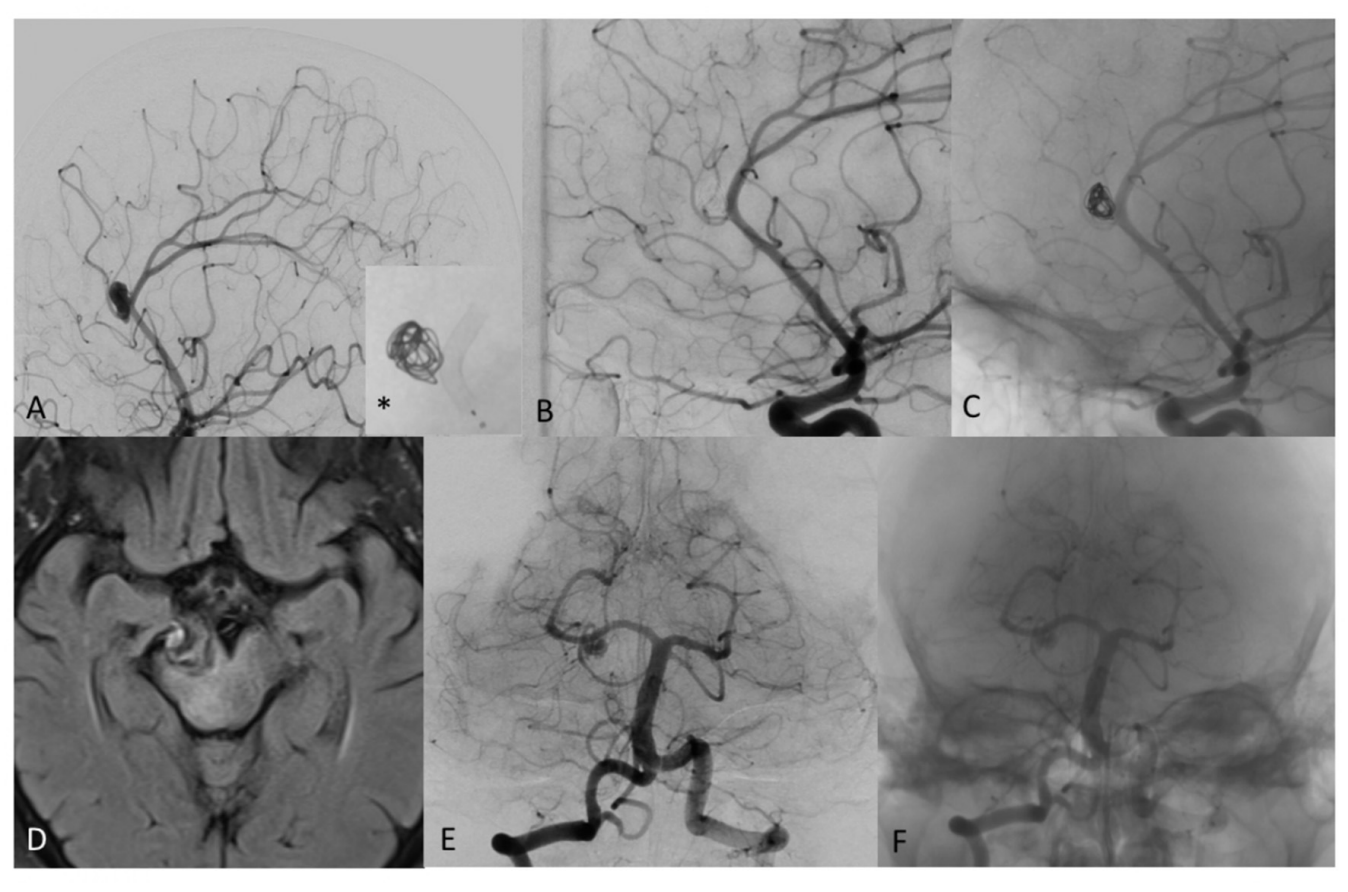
(A) Digital subtraction angiography (DSA) of a patient with distal pericallosal-callosomarginal aneurysm treated with non-compact coiling and FD (*), to maintain the patency of callosomarginal artery arising from the neck aneurysm. (B) DSA shows complete occlusion of aneurysm sac at 6-month follow-up (stent diameter: 2.25 mm, length: 15 mm). (C) Un-subtracted image where it is possible to see the stent and the flow of contrast with the coils of the same patient at 6 months showing complete exclusion of the sac, no contrast filling through the coils and regular patency of treated arteries. (D) T2 weighted MRI image of mostly thrombosed giant PCA aneurysm. (E) DSA 2-month follow-up after FD treatment with remnant neck patency in patient with significant clinical worsening. (F) Un-subtracted image where it is possible to see the stent and the flow of contrast in the proximal part of the neck aneurysm.
